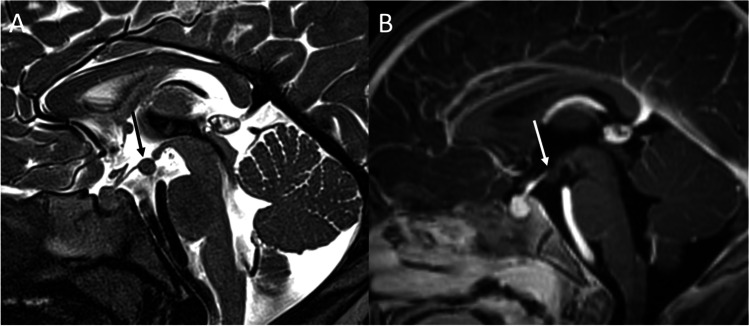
Hypothalamic hamartoma. Sagittal T2- (A) and contrast-enhanced T1-weighted (B) images show a non-enhancing sessile lesion of the tuber cinereum
